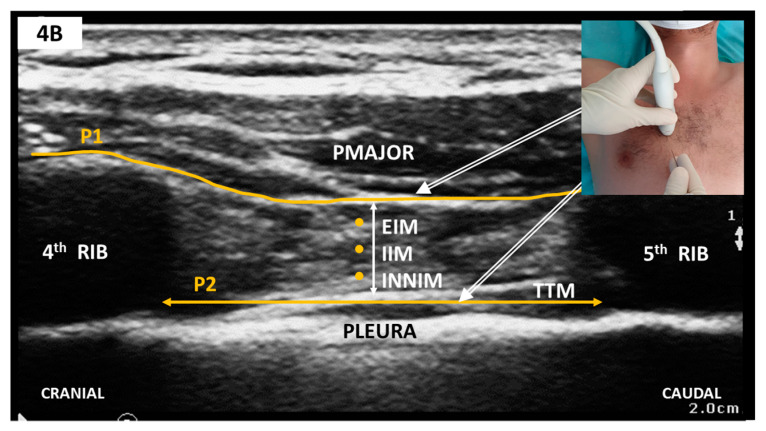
(A) Sagittal parasternal scan; (B) Sagittal parasternal scan with markings. Note that TTM appears as a hypoechoic band folding over the hyperechoic pleura. PMAJOR, pectoralis major muscle; EIM, external intercostal muscle; IIM, internal intercostal muscle; INNIM, innermost intercostal muscle; TTM, thoracic transversus muscle; P1, target plane for PIFB; P2, target plane for TTMPB.
